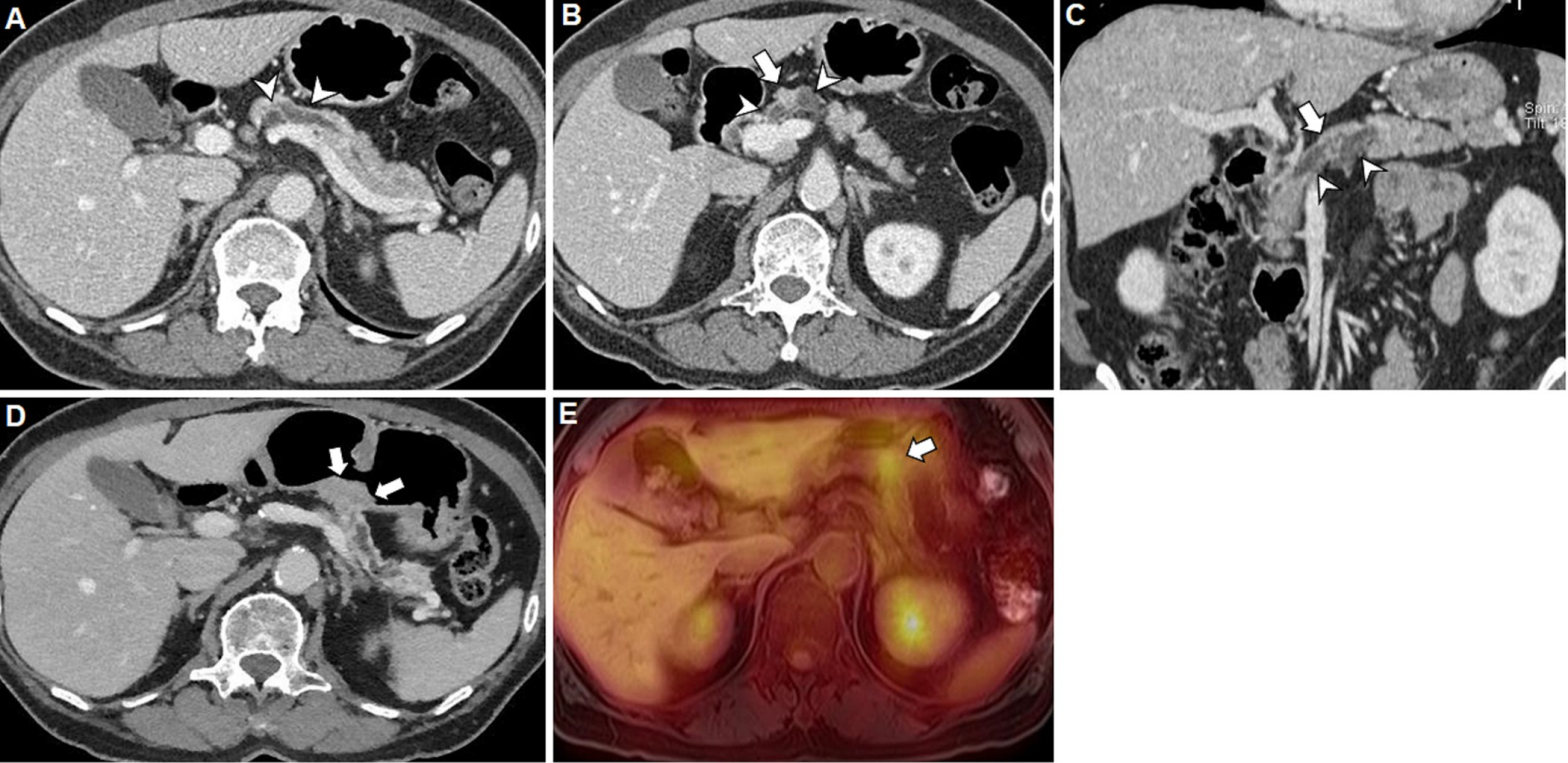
Representative example of CT findings and impact on the remnant pancreas of HG PanIN in a 62-year-old woman with malignant IPMN. A. On the portal phase axial CT image obtained preoperatively, the MPD is dilated up to 8 mm in diameter in the pancreatic body (arrowheads), suggestive of main duct-type IPMN. B-C. The portal phase axial (B) and coronal (C) preoperative CT images show a 9-mm enhancing mural nodule (arrow) within the MPD, and both the downstream and upstream ducts are dilated (arrowheads). The patient underwent central pancreatectomy for the lesion. D. The portal phase axial CT image obtained 5 years after the surgery shows an enhancing soft tissue lesion at the pancreaticogastrostomy site of the remnant pancreas (arrows), which had grown in size on the recent serial follow-up CT. E. On the axial 18F-fluoro-deoxy-glucose (FDG) positron emission tomography (PET)/ MR image obtained three weeks later, the soft tissue lesion at the resection margin of the remnant pancreas shows FDG uptake (arrow). The patient underwent completion distal pancreatectomy, and the lesion was confirmed as a recurrent adenocarcinoma.
